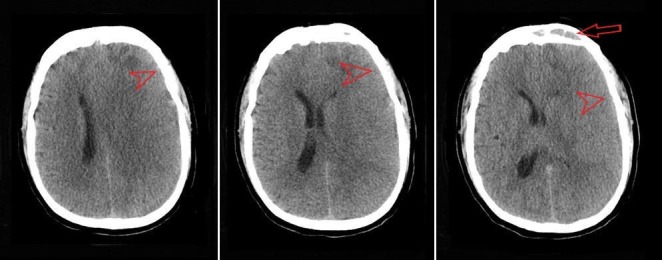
Non-contrast computed tomography (CT) showing possible subacute left subdural hematoma (arrowhead) and suggesting frontal sinusitis (arrow)
